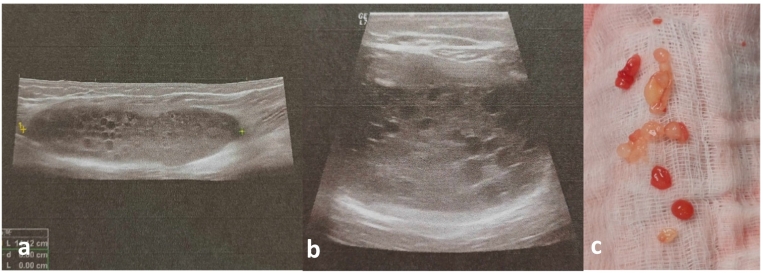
a.b: Ultrasound of the posterior aspect of the thigh showing an intramuscular formation with a well-defined wall containing rounded fluid-filled structures resembling daughter cysts of a hydatid cyst. c: Daughter hydatid cysts corresponding to the ultrasound image of rounded intracystic liquid formations.
